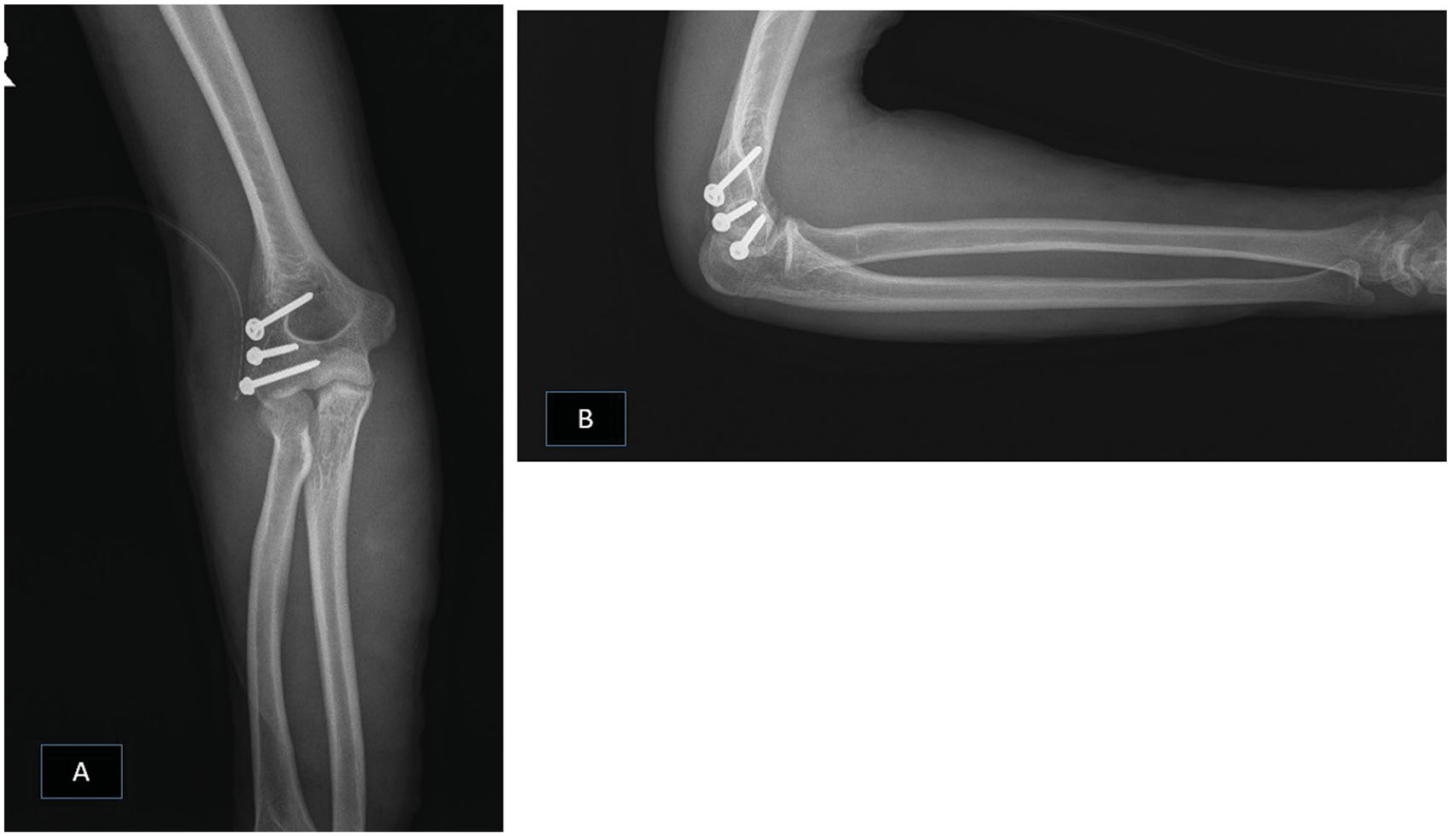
Post-operative AP (A) and lateral (B) radiographs of the patient.
